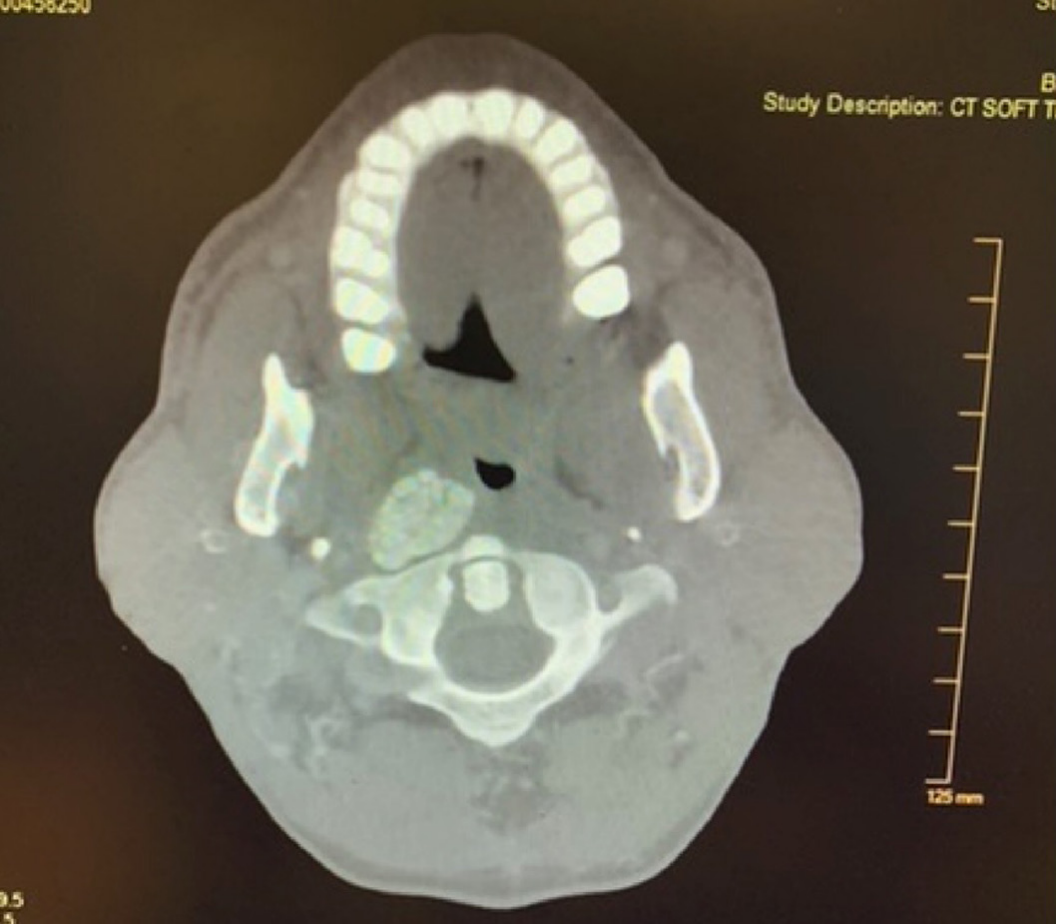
Axial view, CT soft tissue neck. An amorphous calcified soft tissue mass lesion
